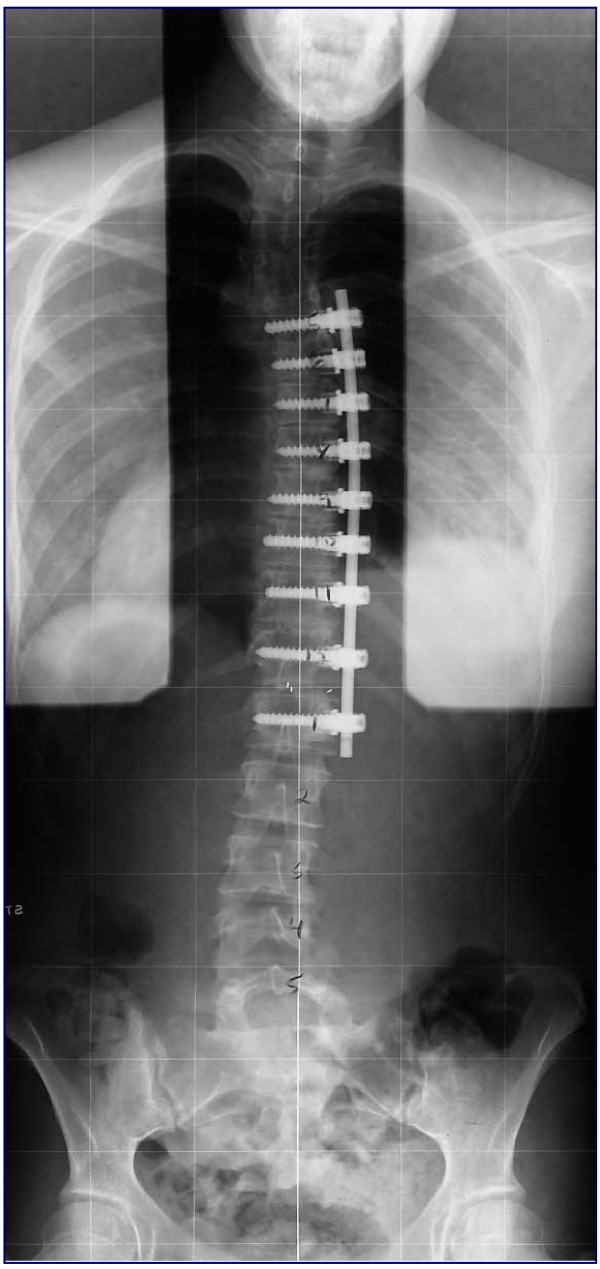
Post-operative plain standing radiograph following thoracoscopic anterior spinal fusion for progressive adolescent idiopathic scoliosis.
